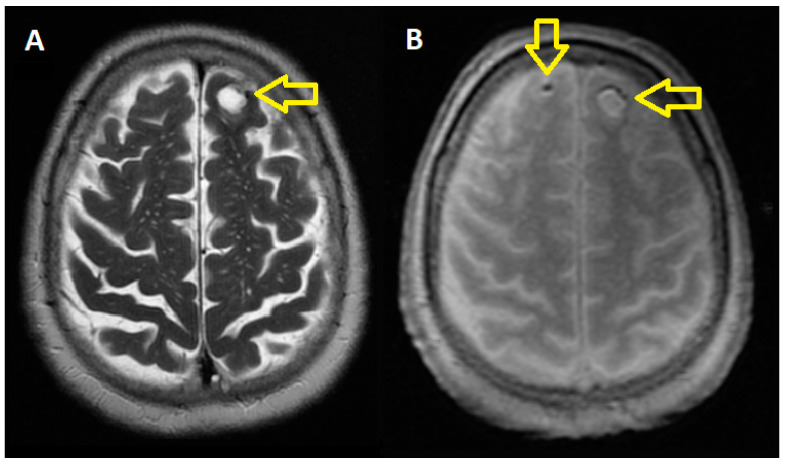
Brain Magnetic Resonance Imaging (July 2021): On the T2 MRI sequence (A) and the T1 MRI sequence (B), the image reveals a prominent subcortical frontal lesion on the left side, which likely contains melanin and appears to be a secondary determination. Additionally, there are seven small, micro-hemorrhagic lesions found in the cortical-intergyral regions of the frontal lobes, bilaterally, as well as in the right posterior parietal region and at the level of the right thalamus. These lesions may be indicative of either cortical micro-hemorrhages in a hypertensive context or micro-secondary hemorrhagic determinations. Yellow arrows: Subcortical lesion- the left image and micro-hemorrhagic lesions in the right image.
